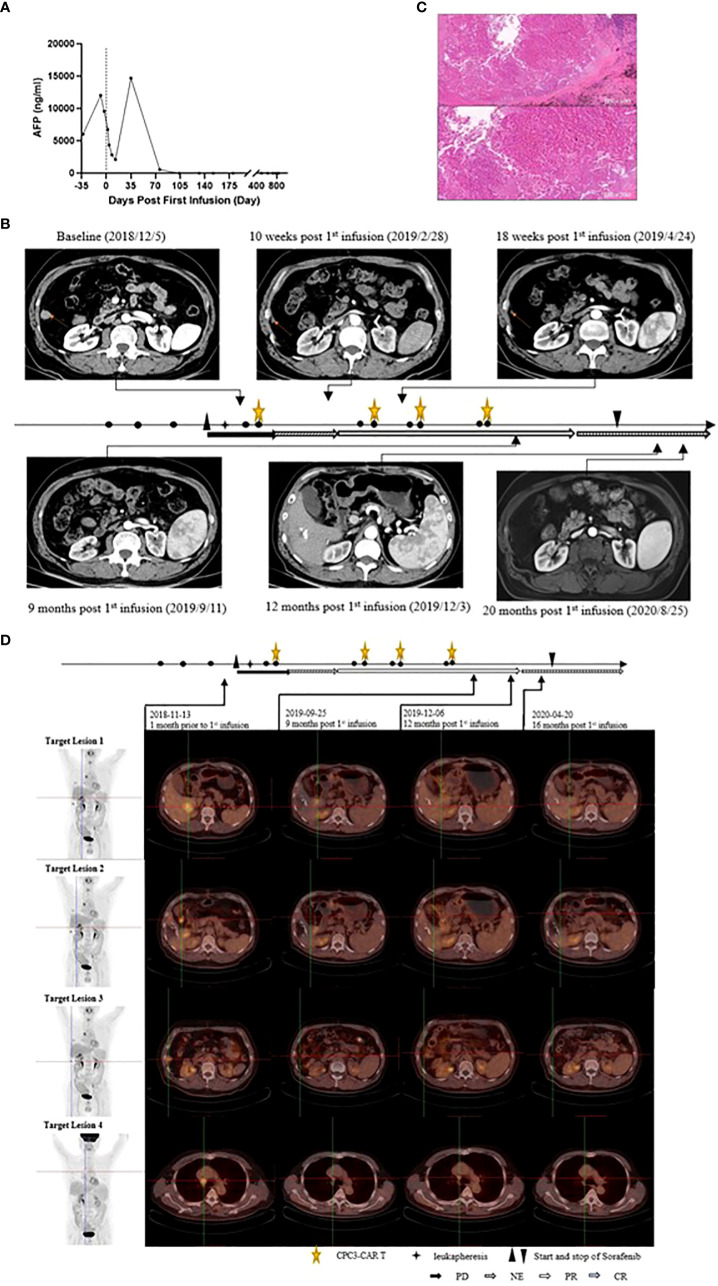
Efficacy of the combination of Sorafenib plus CT011 with lymphodepletion. (A), Changes of the AFP after Sorafenib monotherapy and the initiation of GPC3-CAR T infusion; (B), Changes of the No.3 target lesion. CT scans showed this target lesion was about 16.76 mm at baseline and regressed gradually after the combination treatment. At 9^th^ month, this lesion completely disappeared without relapse; (C), The postoperative pathology picture of the No.4 target lesion at 12th month (HE×100 and HE×200, the pictures showed large patch of coagulative necrosis, peripheral fibrosis, chronic inflammatory cell infiltration, no obvious tumor residue, indicating reaction post anti-tumor treatment); (D), Positron emission tomography-computed tomography (PET-CT) images of the target lesions. The patient received PET-CT check at baseline, 9 months, 12 months, and 16 months post first infusion. No.1, No.2 and No.3 target lesions disappeared at the 12^th^ month, while No.4 target lesion was inactive and was considered as non-specific uptake after anti-tumor treatment.
